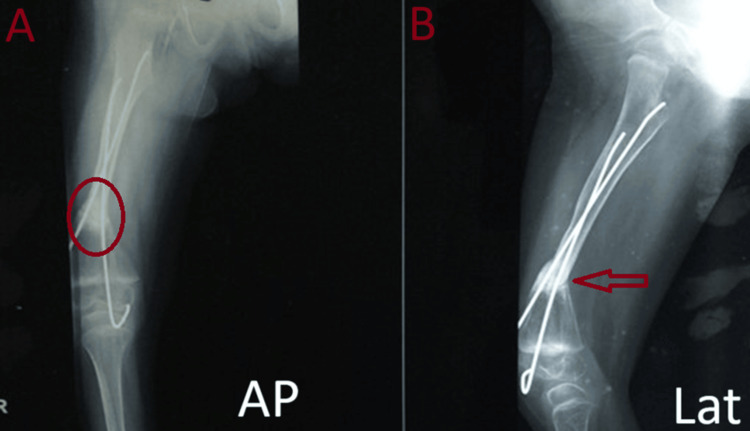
Eighth-month follow-up radiographs showing a progressive sign of fracture union A: AP view, B: Lateral view
